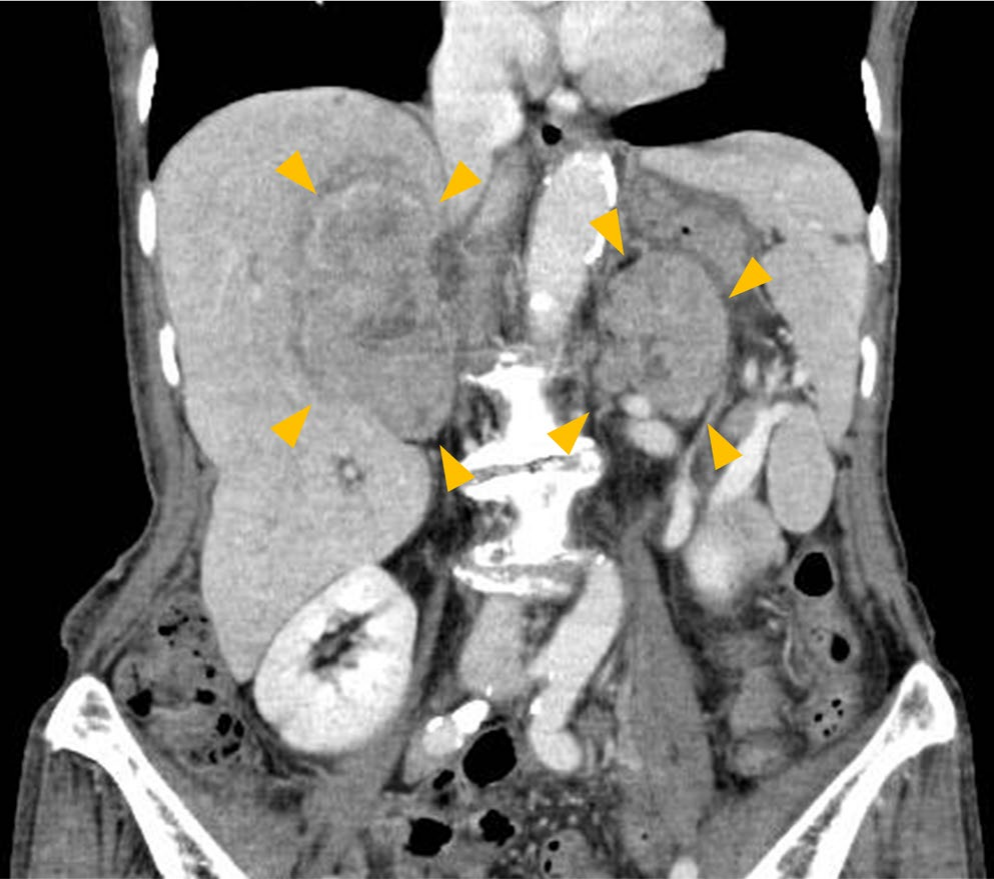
Abdominal contrast‐enhanced CT shows bilateral adrenal enlargement with heterogeneous enhancement.
